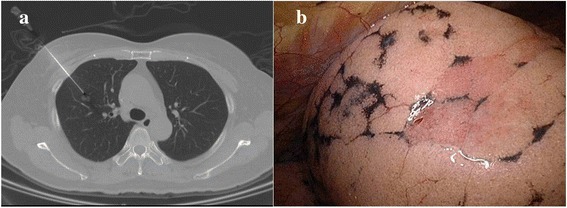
a The puncture needle (Temno Coaxial Introducer Needle, Care Fusion, PP1910) was inserted under the CT-guided imaging. b Intraoperative identification of the puncture holes at lung surface after one lung ventilation
